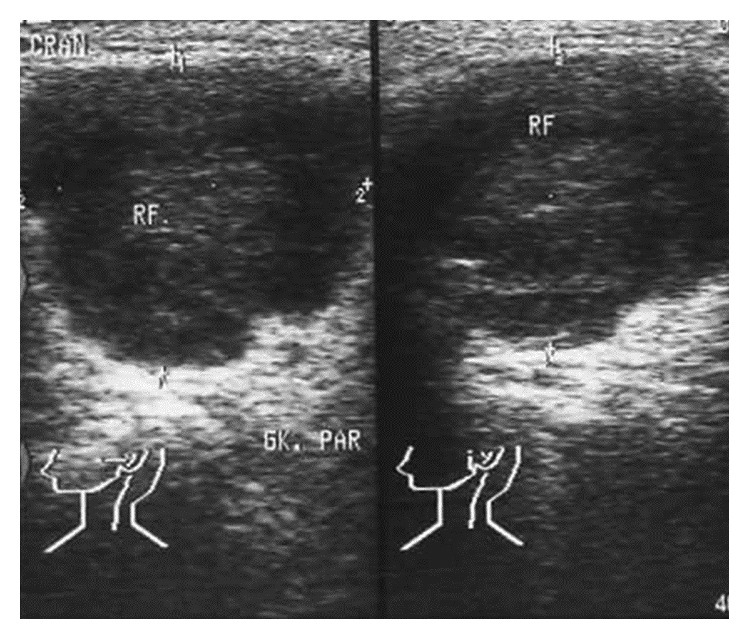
Ultrasonography of the left parotid gland.
